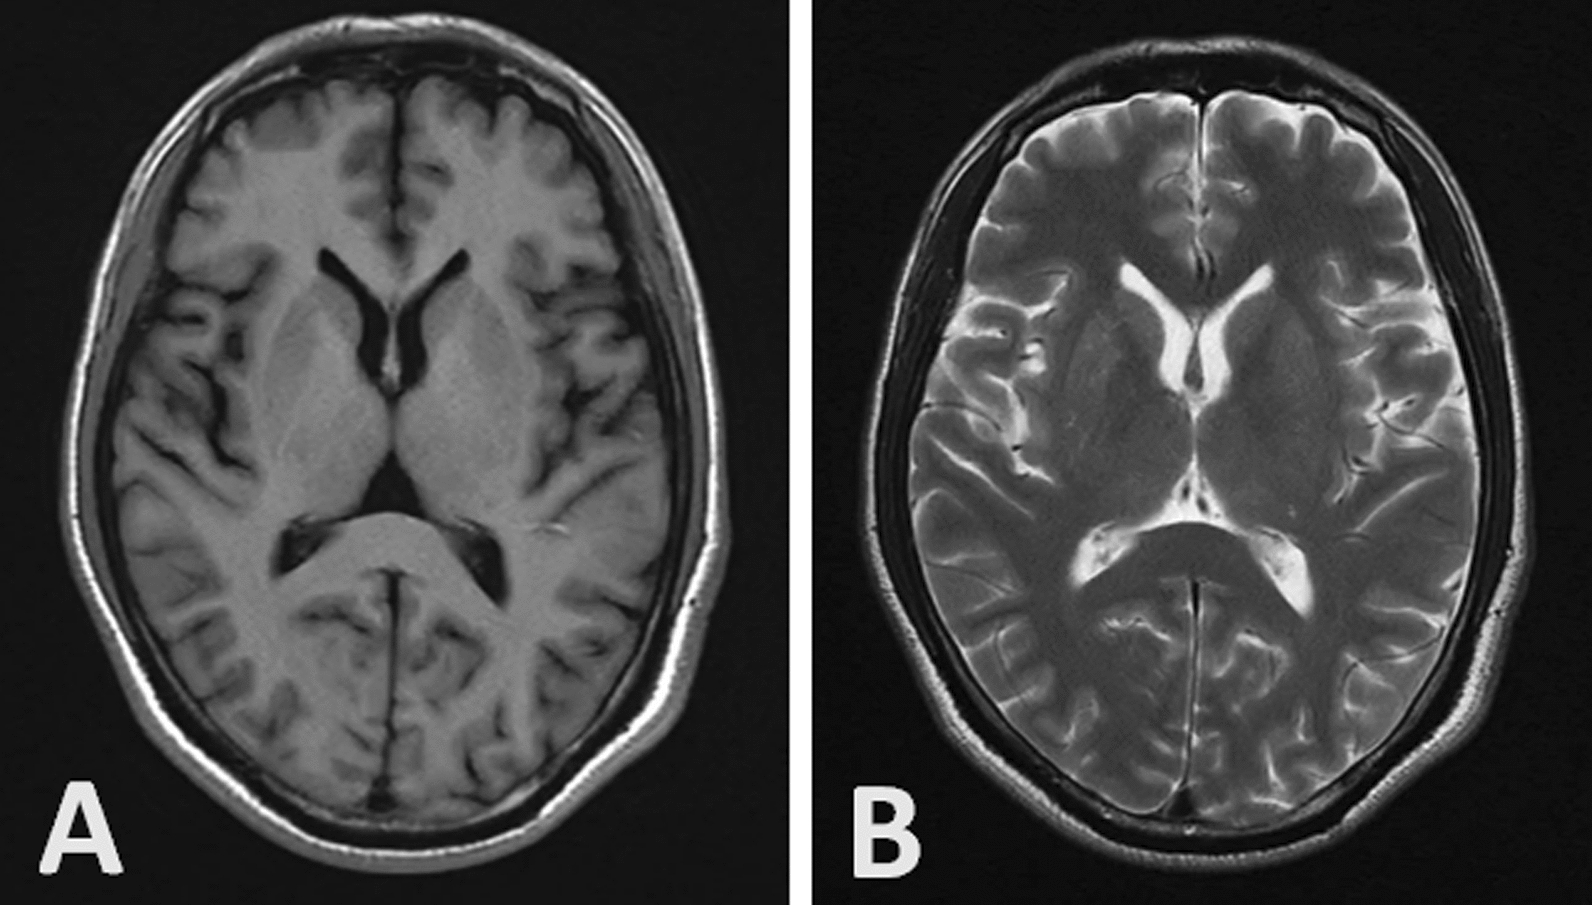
Head MRI of the patient shows no abnormalities in either the T1 (A) or T2 (B) sequence
